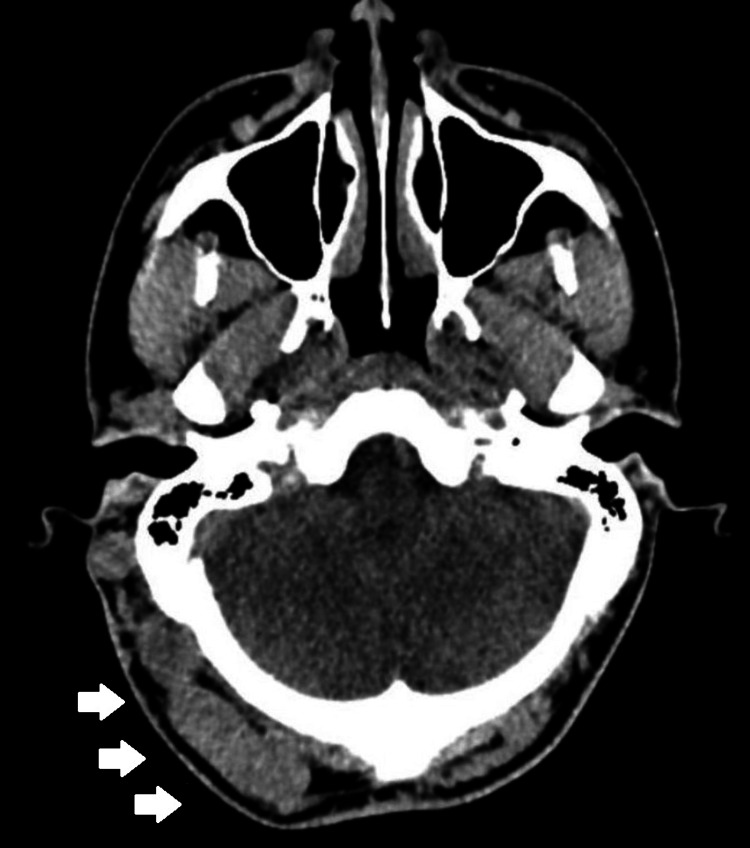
Right occipital EAVF Axial projection of a simple CT showing a soft tissue right parietooccipital mass, later diagnosed as an EAVF. Arrows show an isodense left parietooccipital lesion, which causes an increase in volume EAVF: epicranial arteriovenous fistula
